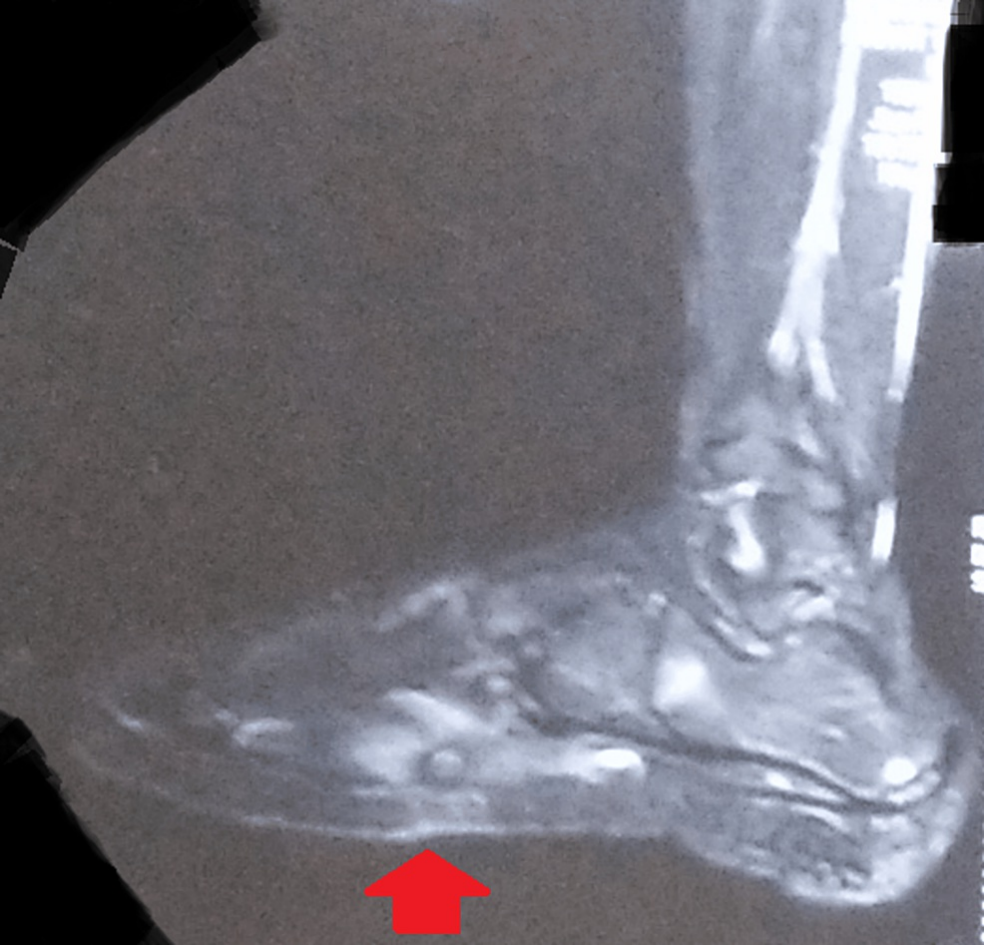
MRI of the right foot showing a hyper-intense sinus tract in the plantar aspect of the midfoot with overlying skin and subcutaneous ulceration with oedema with mild marrow oedema in the fifth metatarsal. MRI: magnetic resonance imaging
